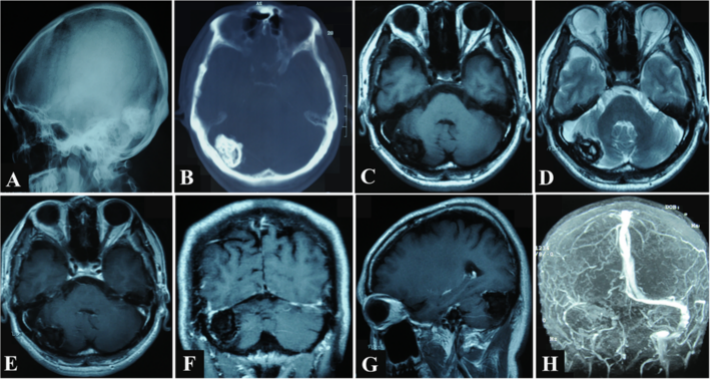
Preoperative radiological images. Normal skull X-ray and unenhanced axial computed tomography (CT) scans display an irregular calcified intracranial lesion in the right occipital region, which is separated from the lamina interna cranii by a narrow cleft. Magnetic resonance (MR) images display a blur nodule in the right transverse sinus and the neighbouring dura. The lesion presents with mixed low and equal signal intensity on unenhanced T1-weighted image and mixed low and high signal intensity on unenhanced T2-weighted image. It exhibits irregular linear contrast enhancement only in its dural periphery after administration of gadolinium. Magnetic resonance venogram (MRV) reveals no detectable venous flow of the right transverse sinus and the right sigmoid sinus. A, Lateral normal skull X-ray films; B, unenhanced axial CT films; C, unenhanced axial T1-weighted MR image; D, unenhanced axial T2-weighted MR image; E, enhanced axial MR image; F, enhanced coronal MR image; G, enhanced sagittal MR image; H, MRV image.
